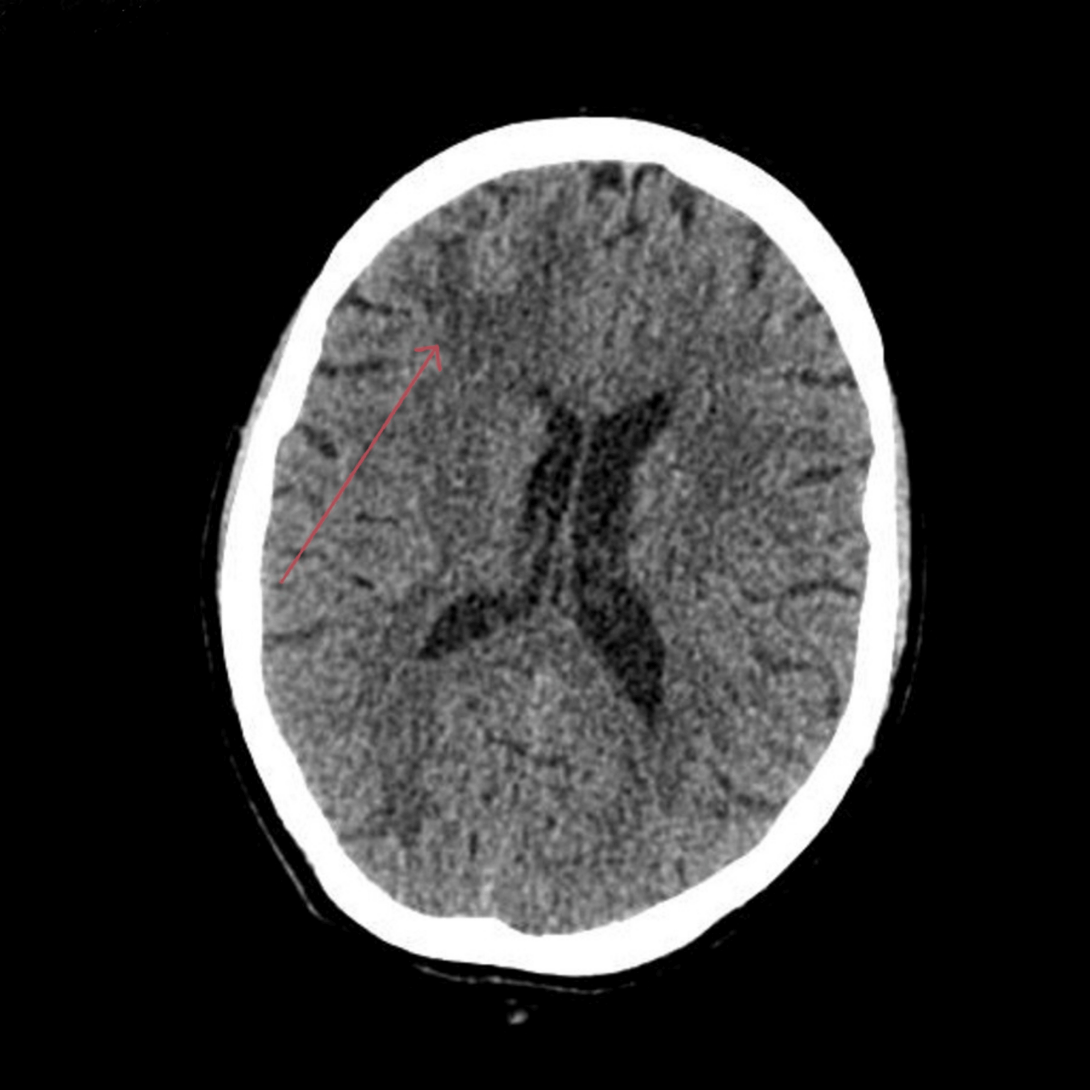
Non-contrast CT head showing asymmetrical right-sided grey-matter hypoattenuation, highlighted by a red arrow.
